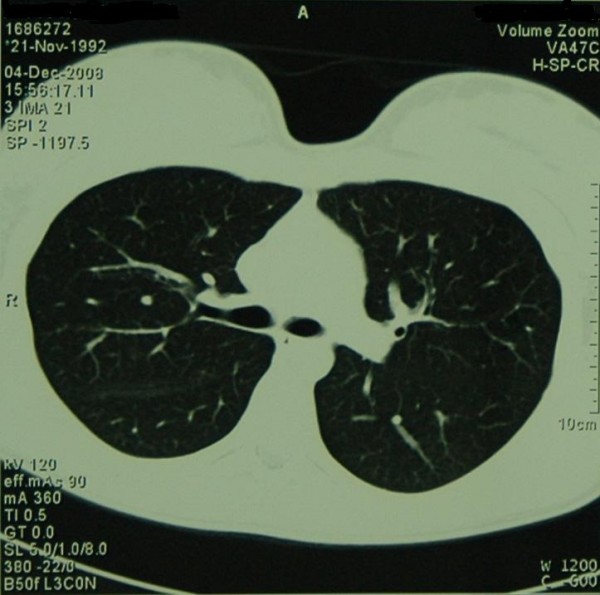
After a three-month period of treatment a new computed tomography scan demonstrated a full recovery.
